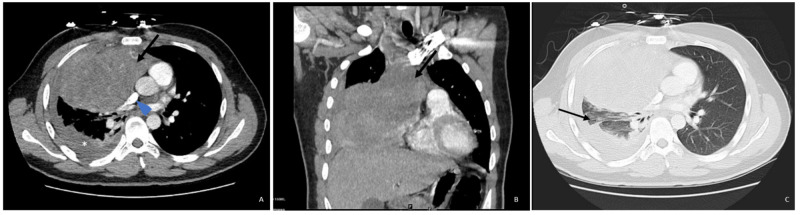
CT Chest Evaluation Axial (A) and coronal (B) contrast-enhanced CT images in soft tissue window demonstrate a large right anterior mediastinal soft tissue mass (arrows) which is largely hypodense with heterogenous enhancement. This mass is effacing the right middle lobe, narrowing of the SVC (arrowhead in A), and causing leftward displacement of the mediastinum. Axial image (A) also demonstrates a small right pleural effusion (asterisk) with adjacent atelectasis. (C) Axial contrast-enhanced CT image in lung window demonstrates the compressive atelectasis (arrow) surrounding the small right pleural effusion to a better extent. A differential based on images and patient’s age includes lymphoma, neurogenic tumor, neoplastic thymoma, or other neoplasms.
